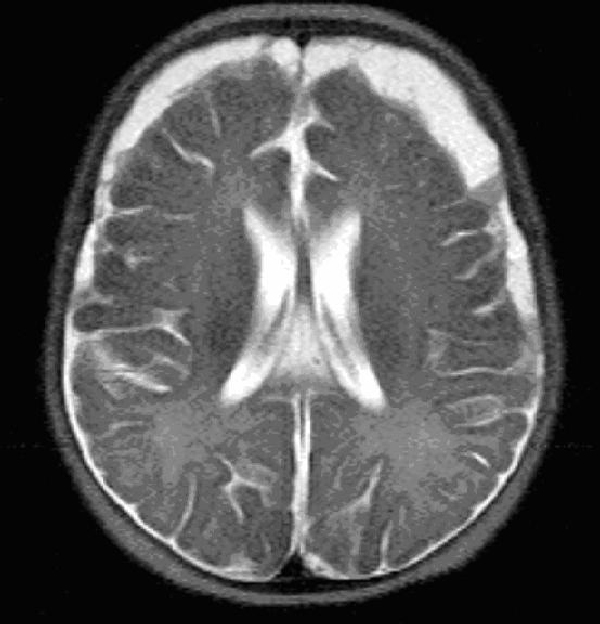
MRI HEAD (axial) without contrast. Meningitis related bifrontal/bitemporal subdural empyema. Notice the pronounced thickening up to 1.2 cm in the left side.
